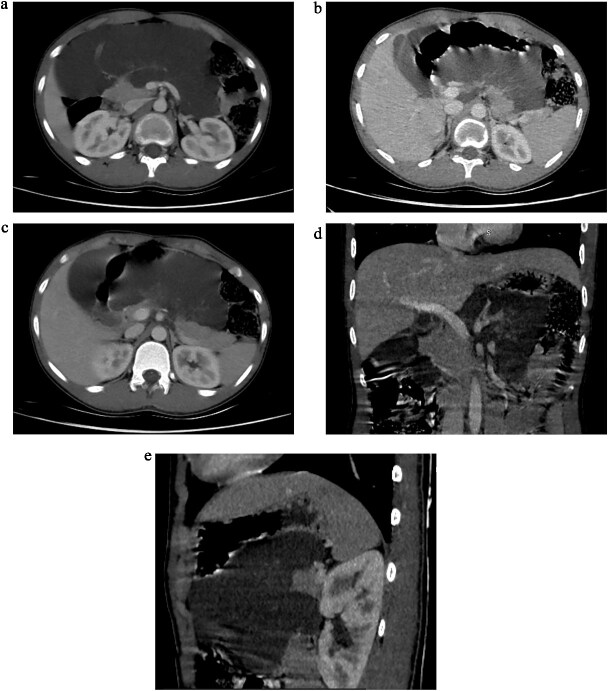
(a) Axial sections of CT abdomen (arterial phase) showing cystic mass in relation to pancreas. (b) Axial sections of CT abdomen (arterial phase) showing cystic mass in relation to pancreas. (c) Axial sections of CT abdomen (venous phase) showing cystic mass in relation to pancreas. (d) Coronal section of CT abdomen (venous phase) showing cystic mass in relation to body and tail of the pancreas. (e) Sagittal section of CT abdomen (venous phase) showing cystic mass in relation to body and tail of the pancreas.
